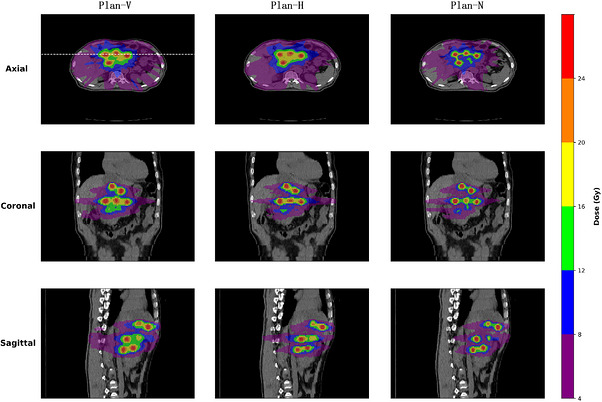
Comparison of dose distributions on computed tomography (CT) images. The images from left to right represent the Versa HD (plan‐V), Halcyon (plan‐H), and NeuRT Aurora (plan‐N) plans, respectively. From top to bottom, the axial, coronal, and sagittal views are displayed. The white dashed lines in the first subfigure indicate the sampling locations of the profiles shown in Figure 2.
